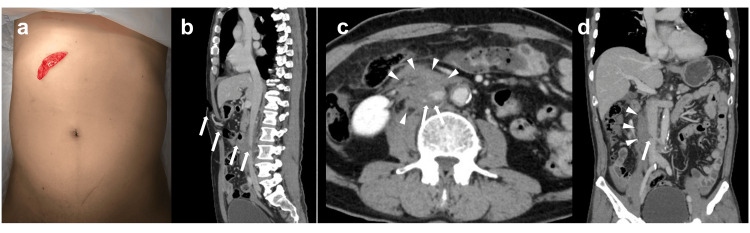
Photographs of the wound and computed tomography (CT) findings at the time of admission. a: Upon reducing the exposed mesentery into the abdominal cavity, a 5 cm stab wound was observed slightly below the right costal margin.
